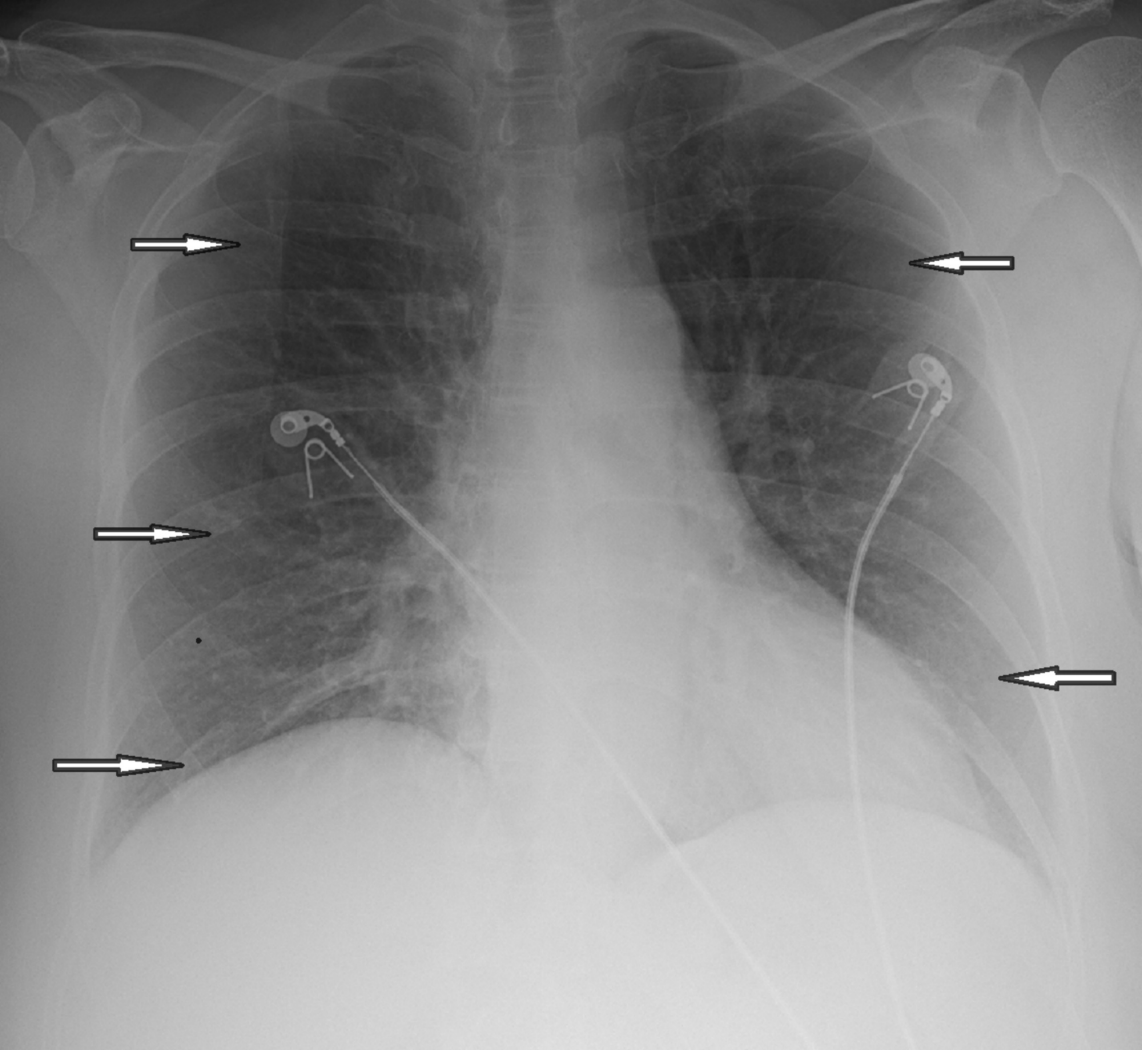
Chest X-ray showing emphysematous changes, no focal consolidation (arrows pointing)
